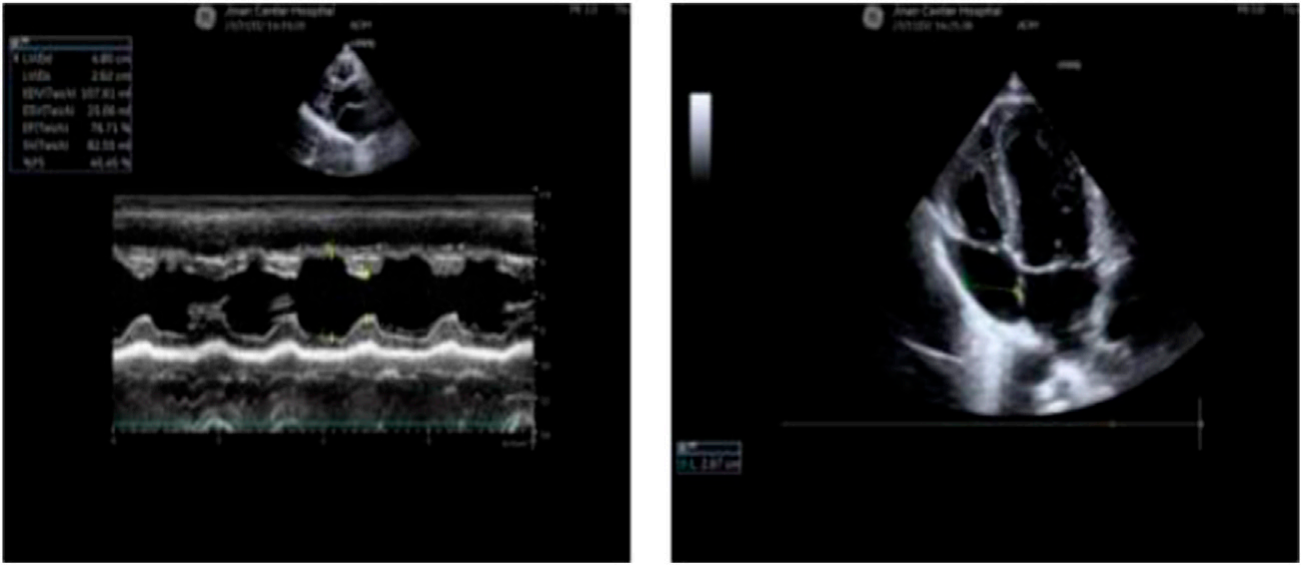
The patient’s cardiac ultrasound revealed the presence of mild tricuspid regurgitation.
